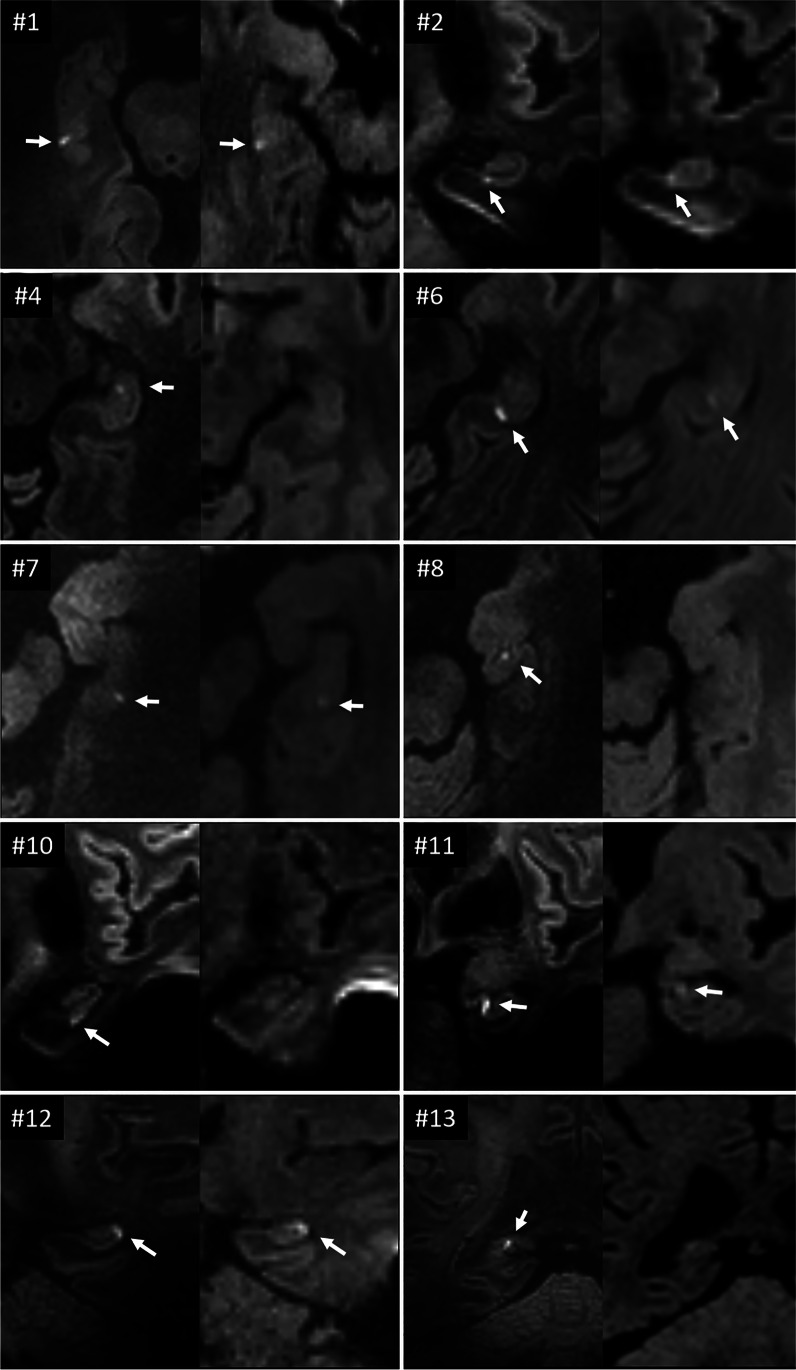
Corresponding DWI-images for 10 of the TGA patients, with the 7 T images to the left and the 1.5/3 T images to the right for each patient. The #-number corresponds to the patient number in Table 1. For patients 4, 8, 10, and 13, the DWI lesion was only detectable at 7 T. For the rest of the patients, the DWI lesions had higher conspicuity at 7 T
